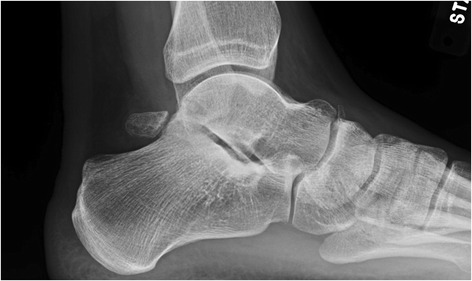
A lateral radiograph demonstrates a large os trigonum
